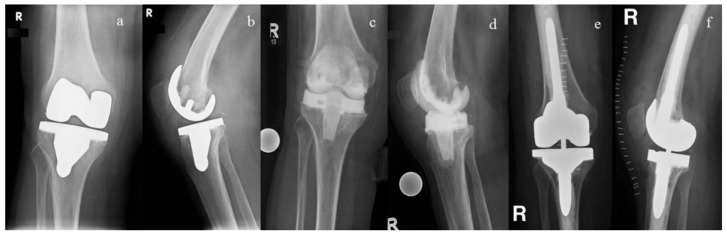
(a,b) Anterio-posterior (a) and lateral (b) radiographs of the right knee of a 69-year-old woman with a septic loosening of the tibial component; (c,d) After prosthesis explantation, an articulating spacer was implanted; (e,f) Following an interim period of 54 days, a condylar-constrained prosthesis was implanted (Triathlon TS^®^, Fa. Stryker, Duisburg, Germany).
